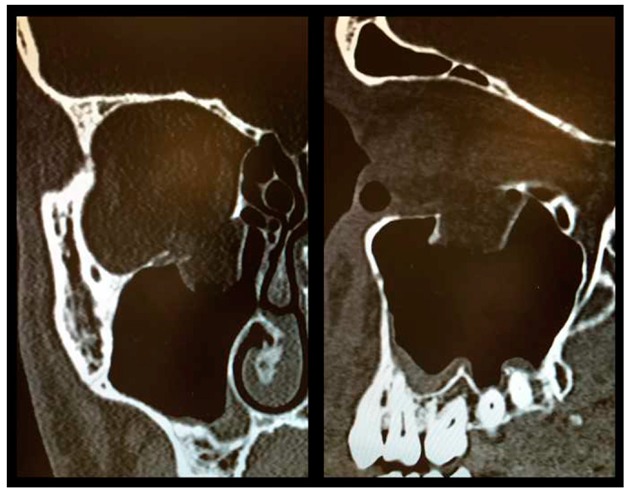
Preoperative CT scan showing a large OFF ((left) coronal view, (right) sagittal view).
